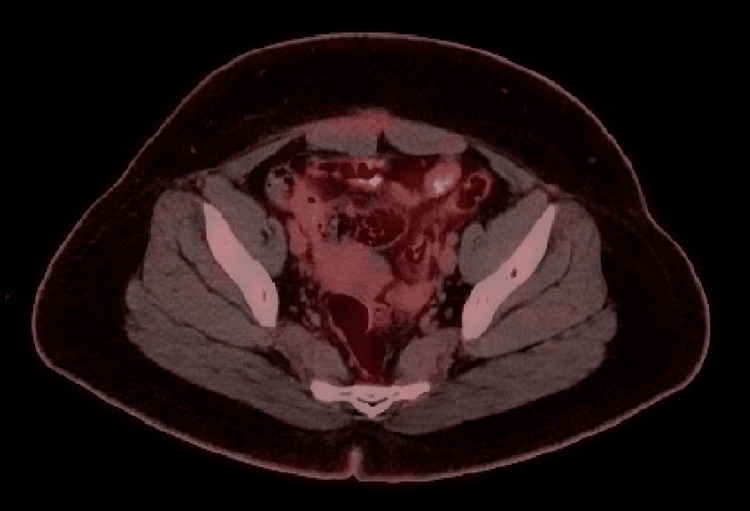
Follow-up PET-CT scan showing no evidence of metabolic disease Follow-up PET-CT scan performed after treatment, demonstrating no pathological radiotracer uptake in the abdominopelvic cavity, consistent with absence of active disease or recurrence.
